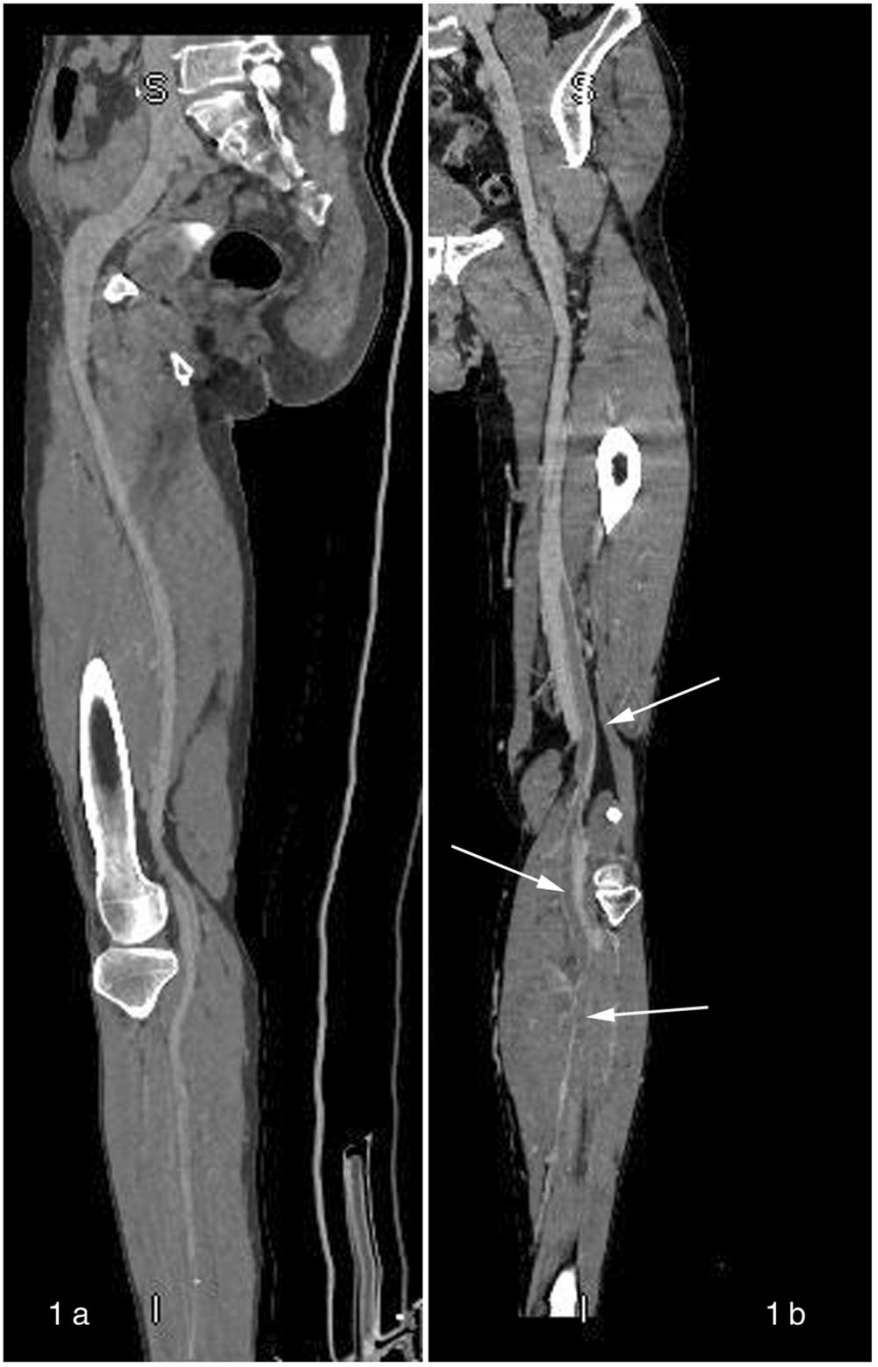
CT venography of normal veins and DVTs. Veins in panel 1a are normal, while multiple DVTs are seen in panel 1b (intraluminal filling defects indicating DVTs are shown by the arrows).
